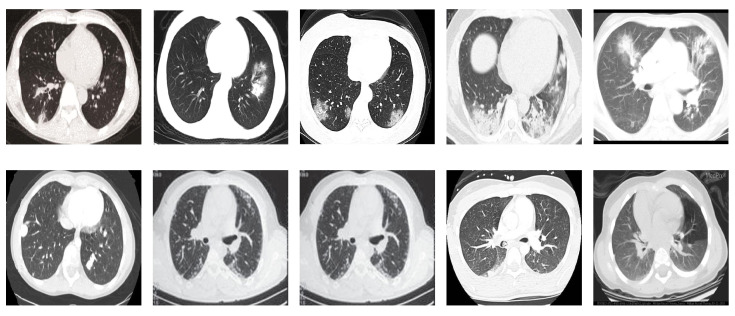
Examples of chest CT images from the COVID19-CT dataset. The first row represents CT images diagnosed with COVID-19, whereas the second row represents non-COVID-19 cases, but other lung diseases.
